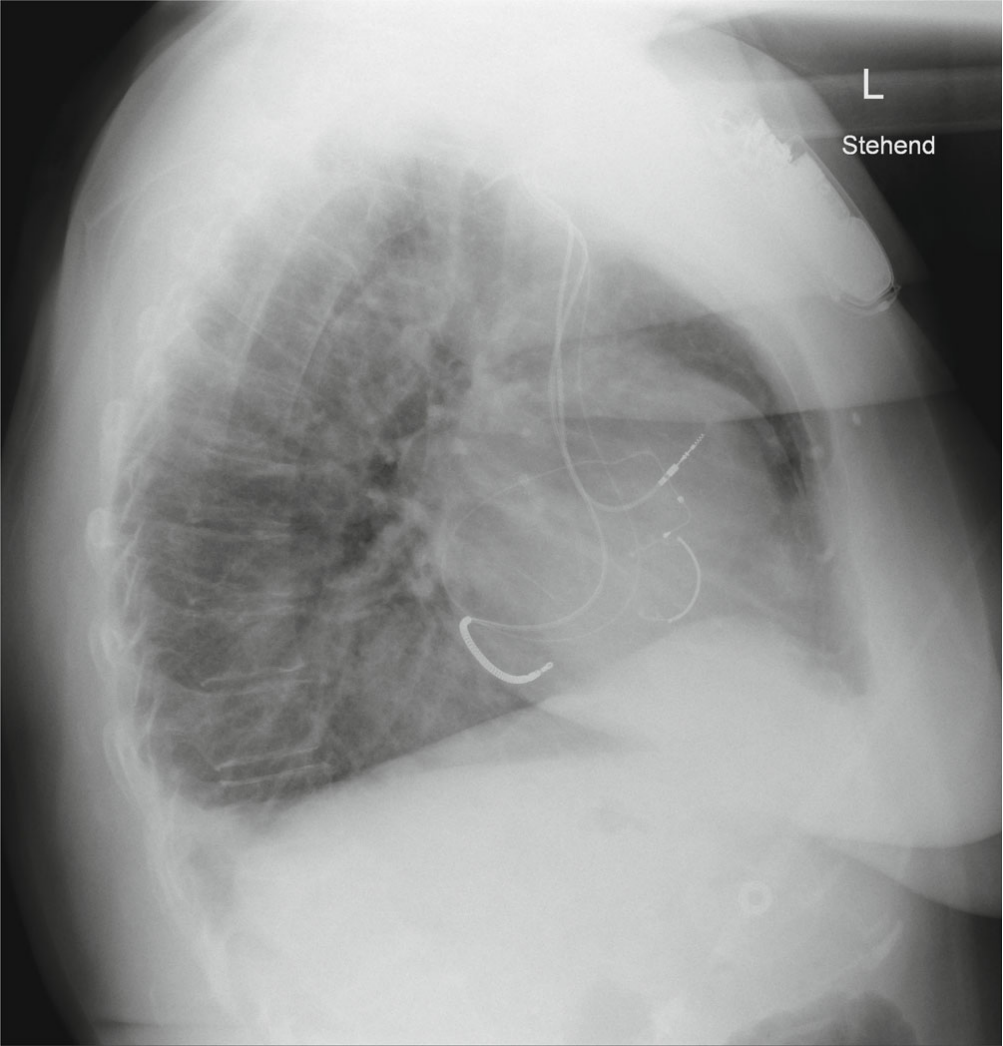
Chest X-ray in lateral view.
